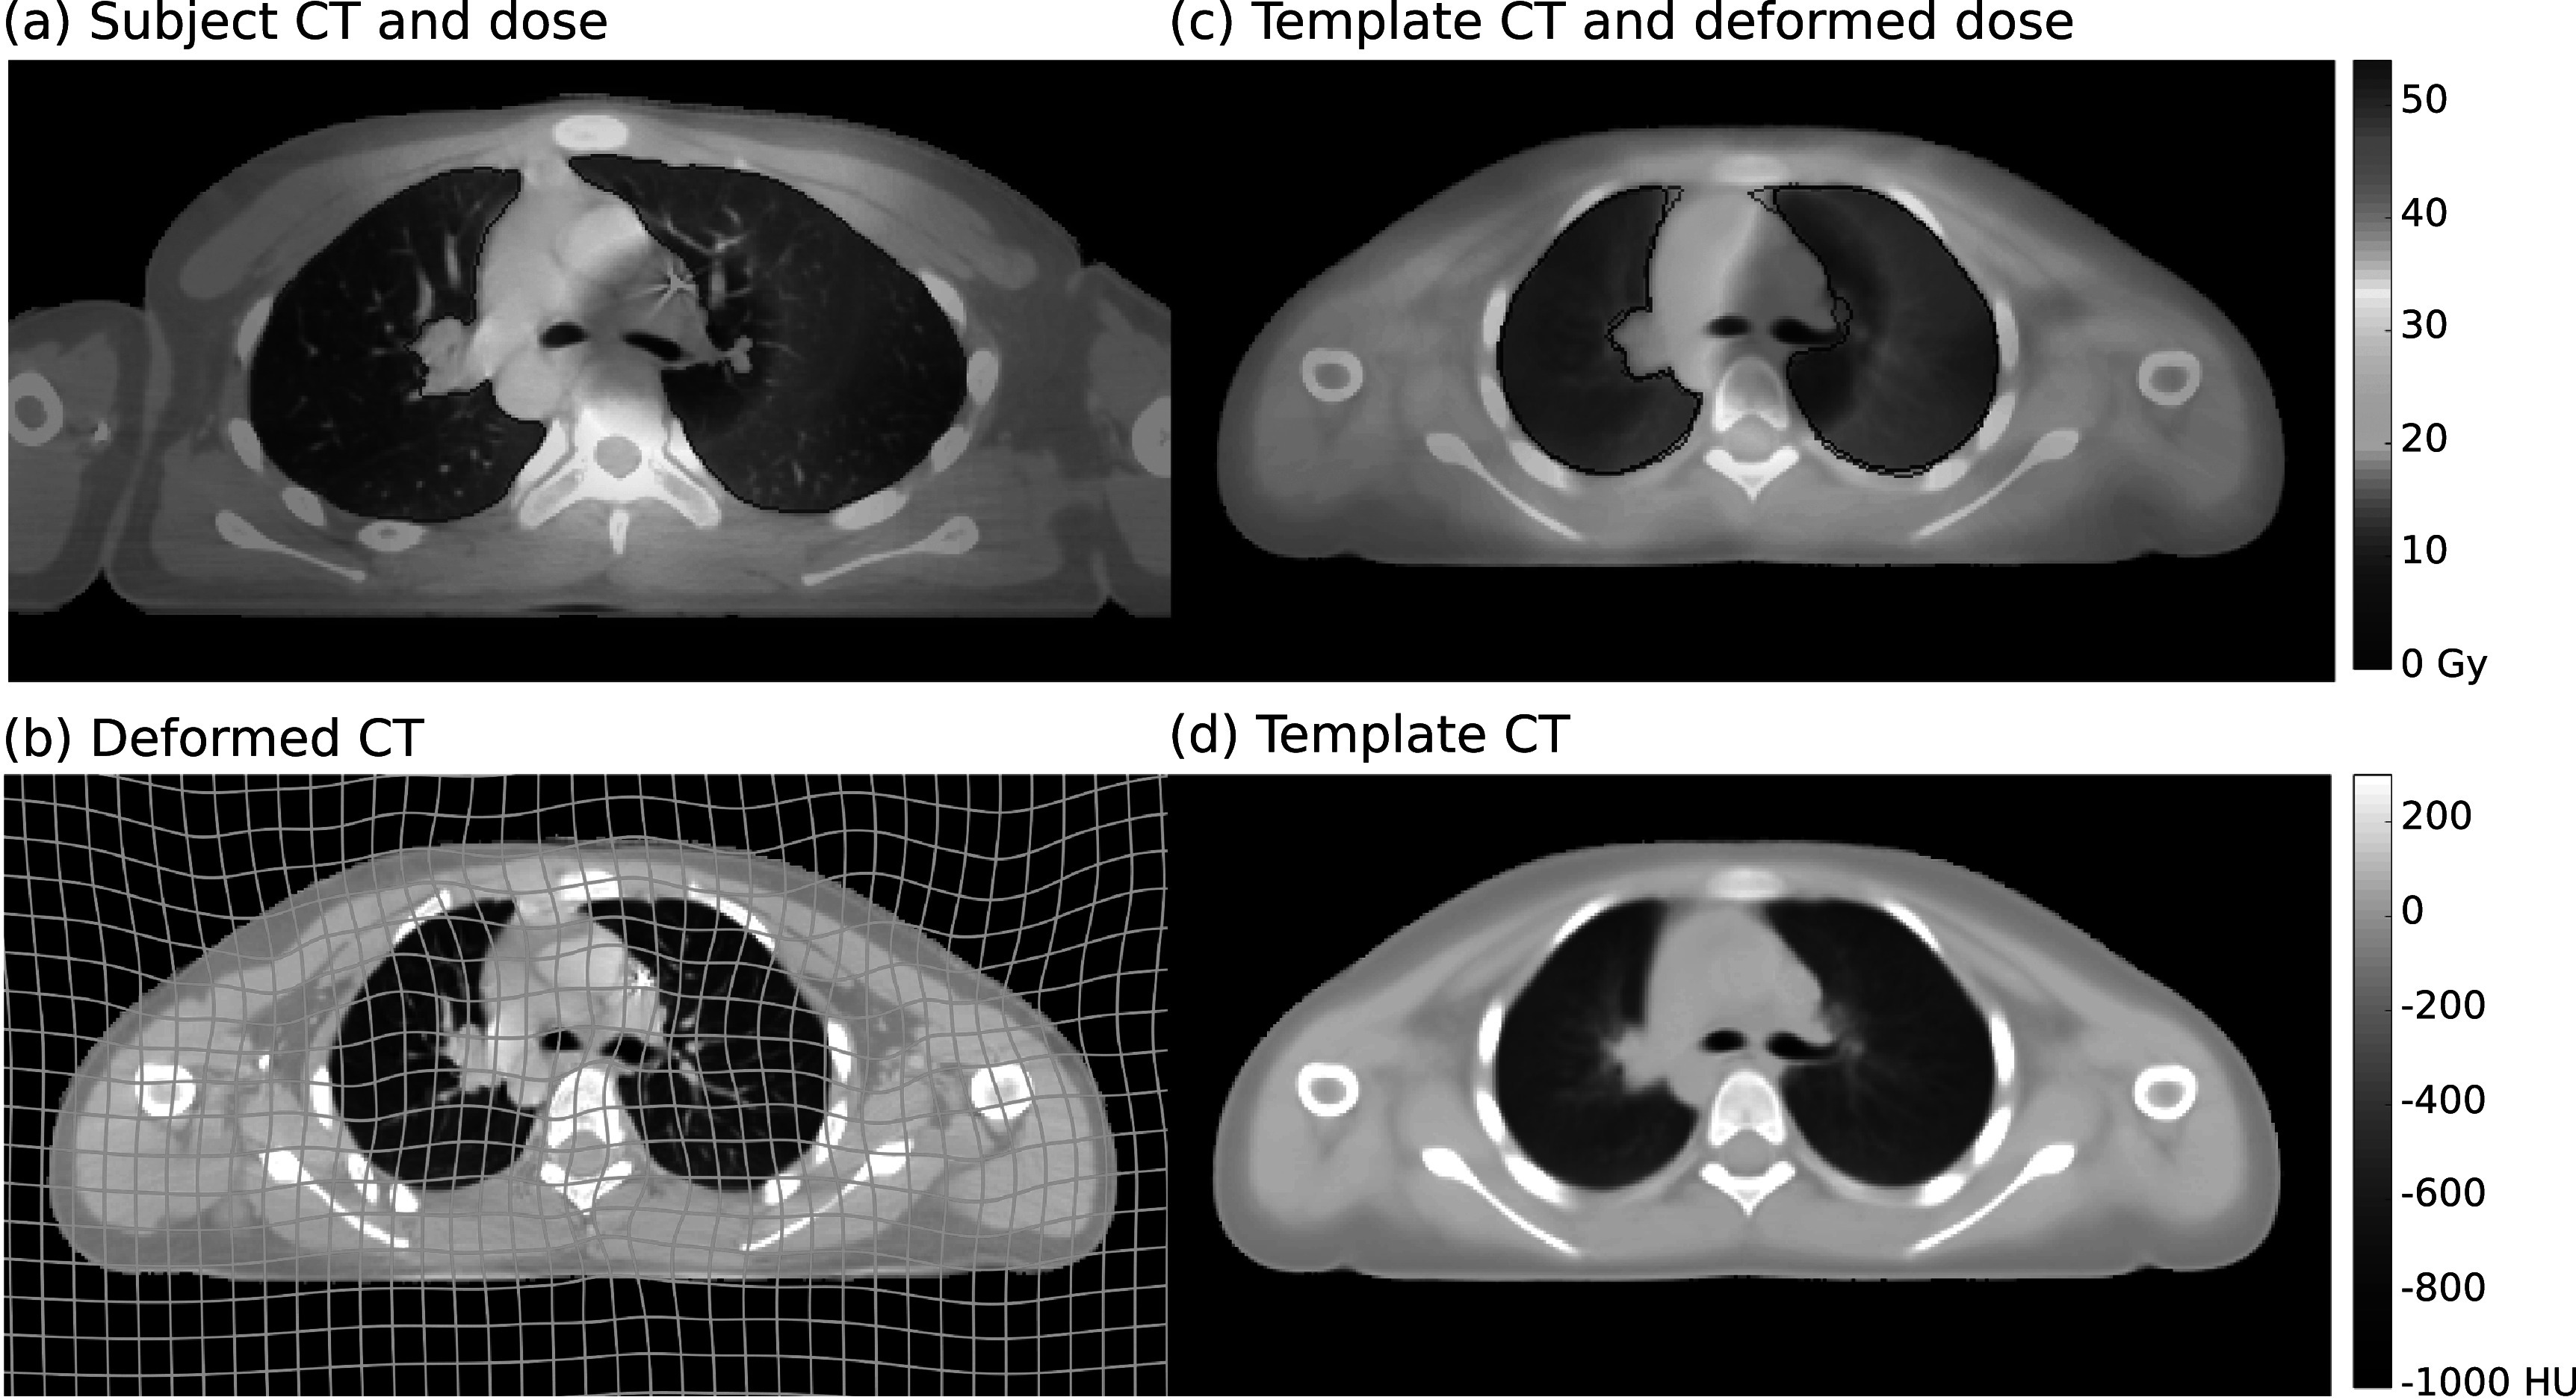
Example of data (images, contours and doses) used in the evaluation experiments. (a) CT, lungs contour (red) and dose distribution for one of the subjects included in the analysis (Ewing sarcoma); (b) subject CT propagated to the template (affine followed by deformable registration), and corresponding deformation grid; (c) template CT and lungs contour (blue), and overlaid deformed lungs contour (red) and deformed dose distribution; (d) template CT by itself.
